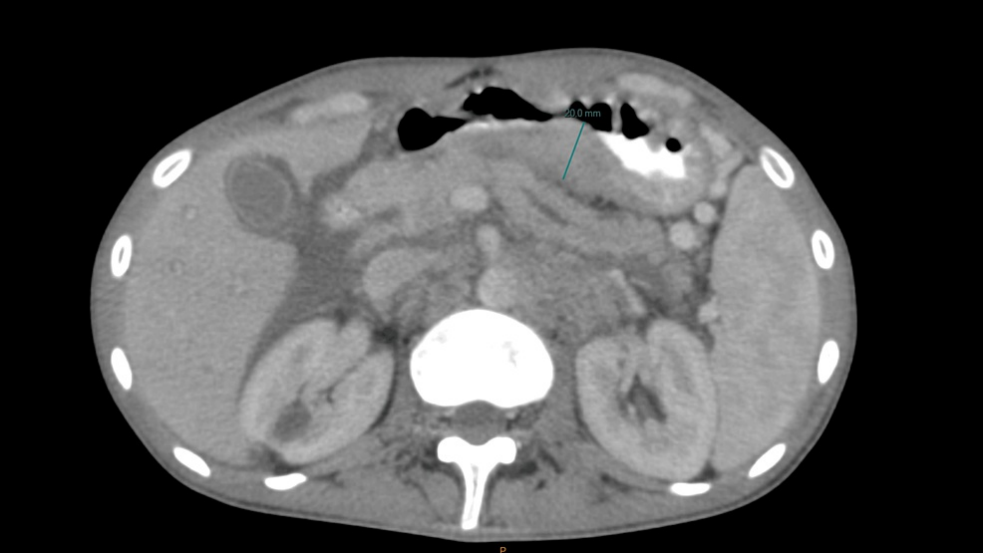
Axial CT scan images Showing the primary gastric tumor located in the posterior wall of the antrum with a thickness of 20 mm.
